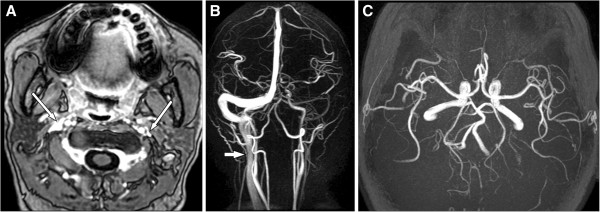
Quantitative grading of IJV compression/stenosis. Contrast-enhanced, axial T1-weighted images of quantitative grading of IJV compression/stenosis between 0 (none) and 3 (severe). Examples at the upper IJV level (C1-C3) demonstrate no stenosis (A) or mild (B), moderate (C), and severe (D) stenosis (arrows).
